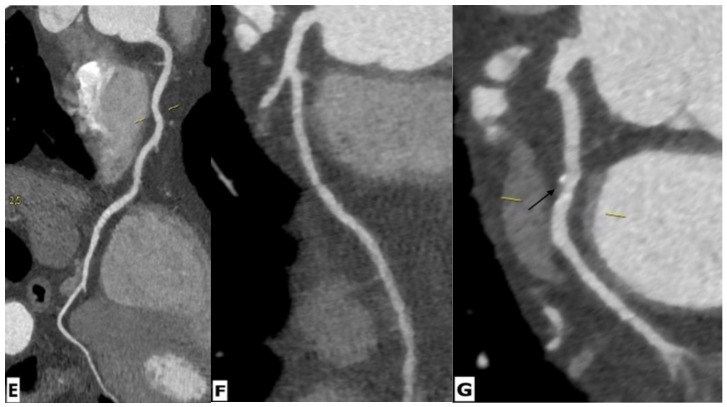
Cardiac magnetic resonance imaging in a 65-year-old HIV-infected male patient with microvascular coronary dysfunction. (A) Mid-ventricular short-axis late gadolinium enhancement image showing no ischemic pattern. Stress CMR was performed via regadenoson administration. (B–D) Stress protocols images of three ventricular slices (basal, mid-ventricular, and apical slices) show diffuse subendocardial hypoperfusion (arrows) due to microvascular dysfunction. (E–G) Multiplanar reformatted contrast-enhanced computed tomography images showing the right coronary artery (RCA), left anterior descending artery (LAD), and left circumflex artery (LCx), respectively. RCA and LAD are normal without any atherosclerotic diseases. LCx shows focal mixed plaque causing minimal luminal stenosis (arrow).
